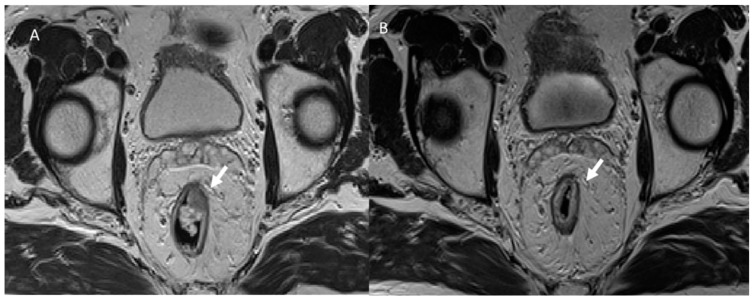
T2-W MRI assessment in pre (A) and post (B) neoadjuvant treatment for rectal cancer. In (A) pre-treatment, the arrow shows a T2 rectal tumor, with the radiological complete response in (B) (arrow).
